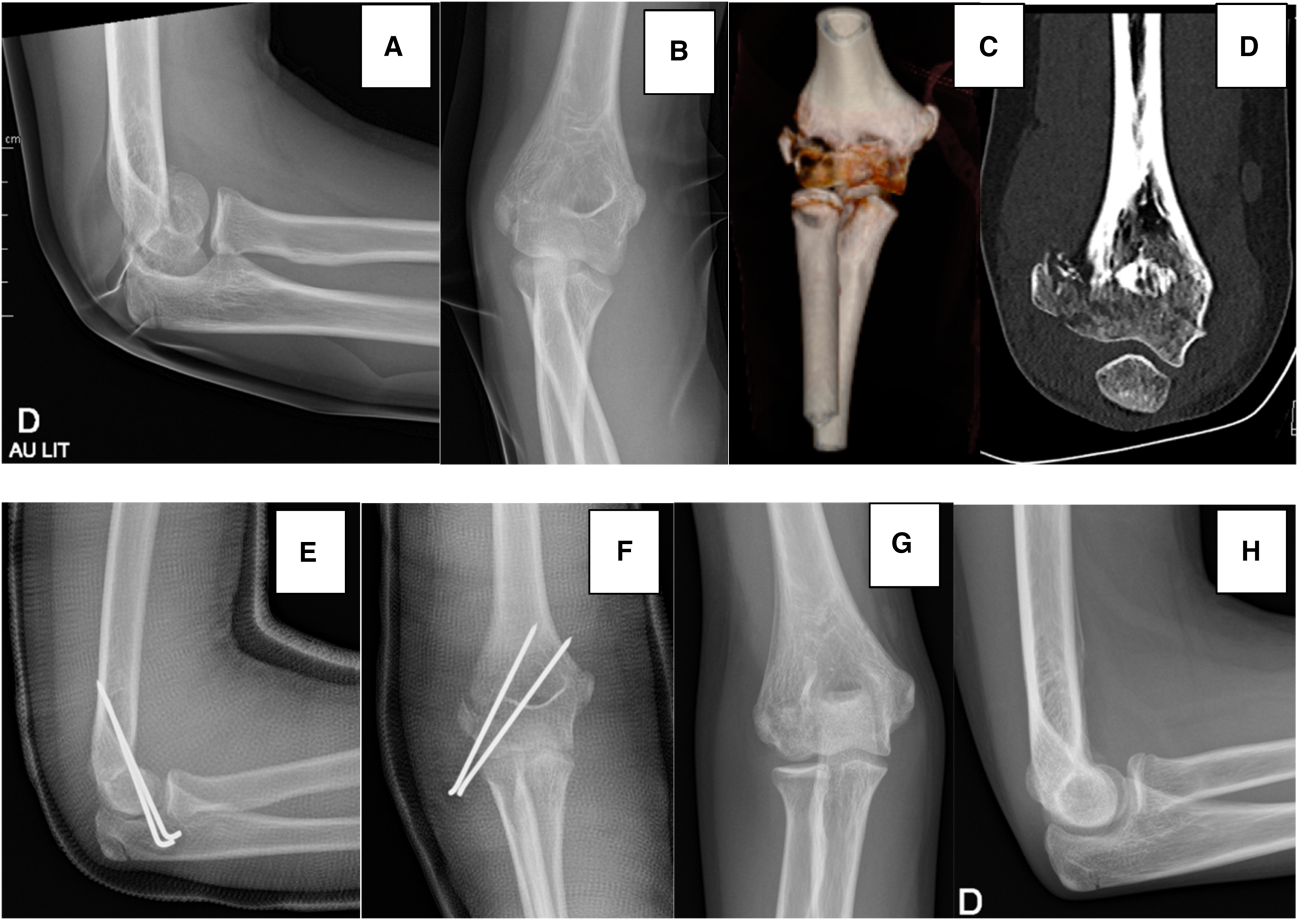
Case 2. (A) Lateral radiograph at admission, showing the double contour sign, (B) AP radiograph at admission. (C) 3D CT-scan showing the extension of the fracture towards the trochlear groove. (D) Coronal slice of the CT-scan showing the lateral displacement of the fragment. (E,F) Postoperative radiographs. (G,H) 2-month follow-up radiographs.
